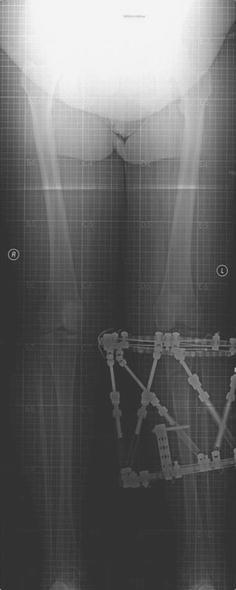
Long leg standing AP radiograph post-correction
